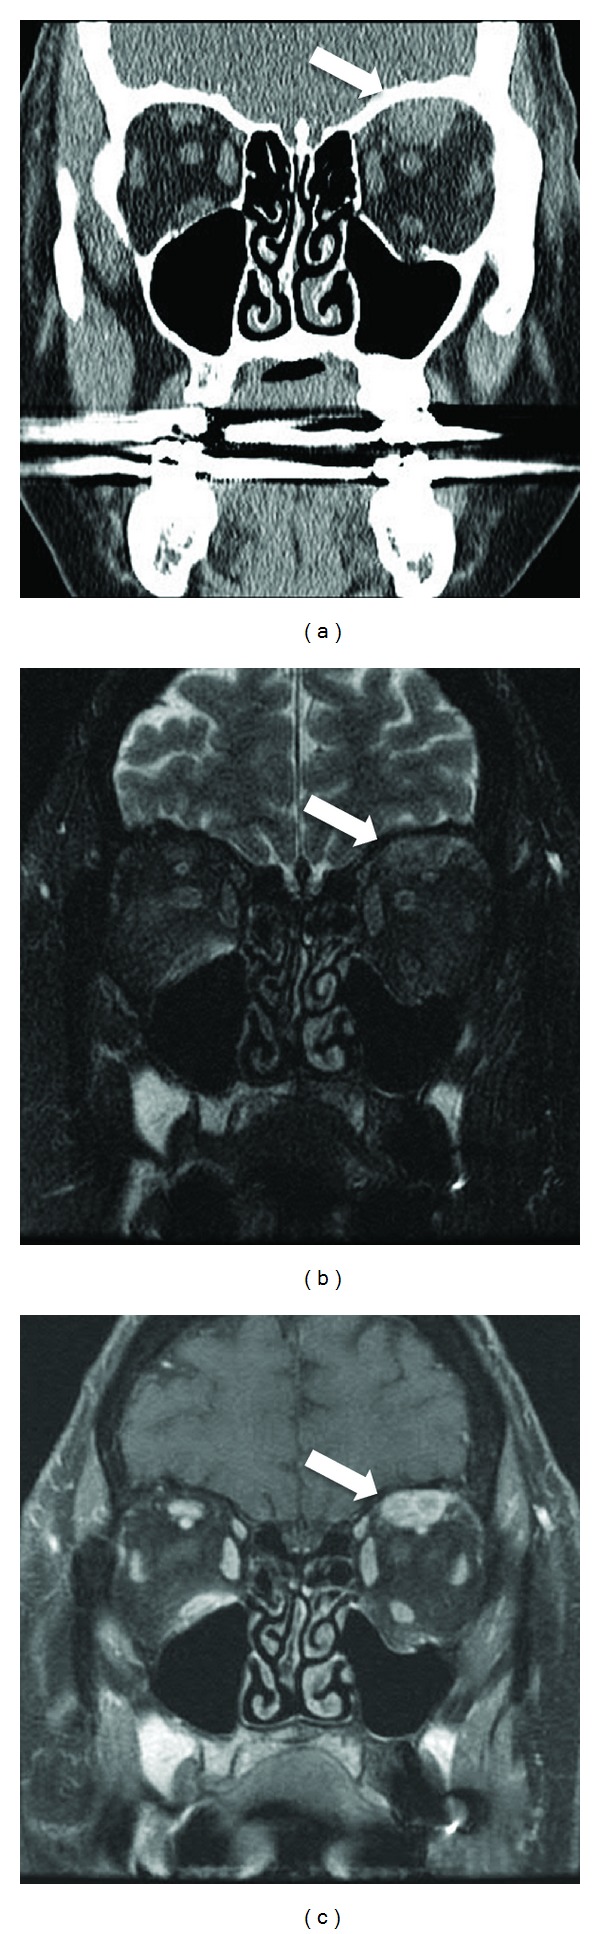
Coronal images from a noncontrast CT (a), fat-saturated T2WI (b), and fat-saturated postgadolinium T1WI (c) demonstrate a noncalcified, avidly though heterogeneously enhancing mass involving the left superior rectus muscle (arrows). Note the lack of any significant intra- or extraconal inflammatory changes in the orbital fat, and the slightly displaced but normal caliber superior ophthalmic vein along the inferior aspect of the mass.
